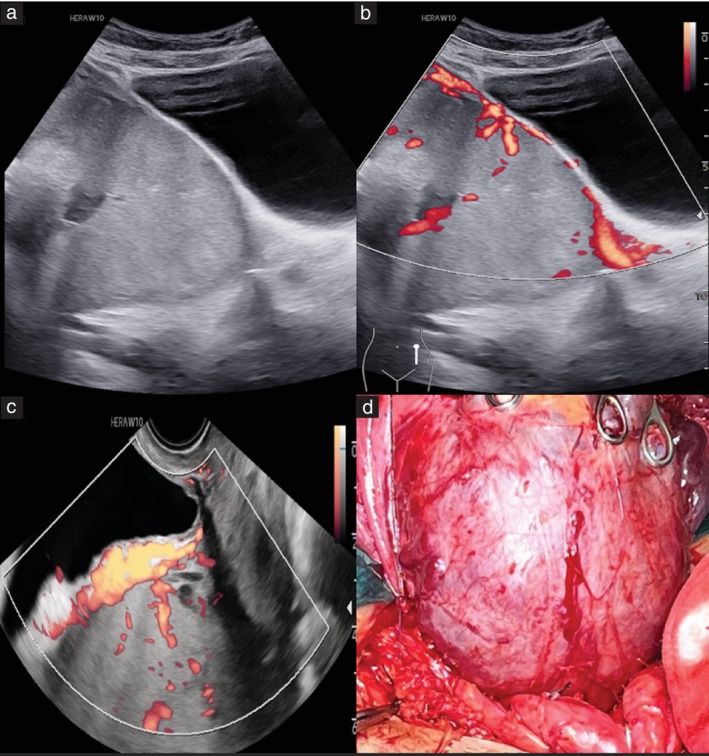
Ultrasound images in a patient with one previous Cesarean delivery and anterior placenta previa. (a) Grayscale ultrasound image showed loss of retroplacental clear zone, absent lacunae and absent bladder wall interruption. Transabdominal (b) and transvaginal (c) color Doppler images showed subplacental hypervascularity. All other abnormal color Doppler features, such as lacunae and serosal hypervascularity, were noticeably absent. (d) The intraoperative image shows an apparently normal lower uterine segment. A diagnosis of low‐grade placenta accreta spectrum was made based on the inability to separate the placenta from the uterine wall, as it remained ‘glued’ to the uterus.
